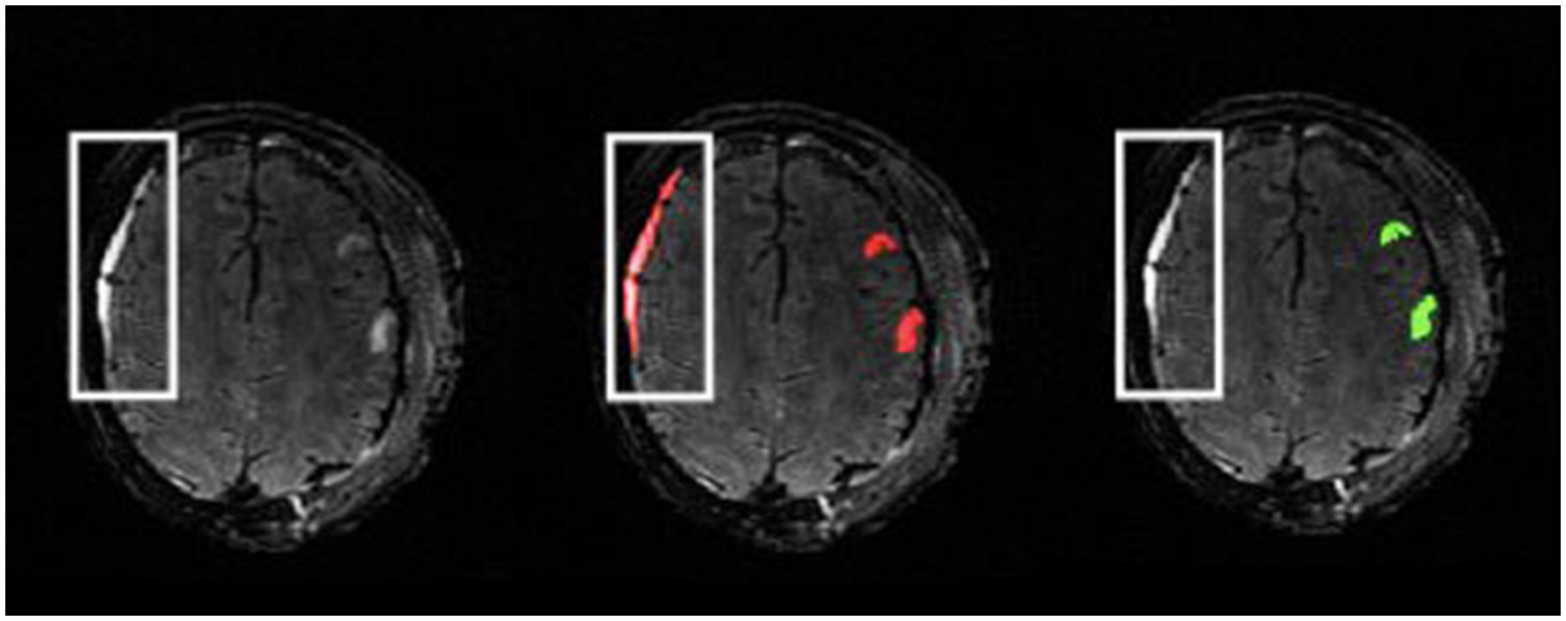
Example of subject with subdural hematoma presenting as a hyperintense region in the outlined area on a T2-FLAIR (left). The initial segmentation includes this as lesion core (middle). The reviewed ground-truth (right) excludes this region from the parenchymal lesion segmentation.
